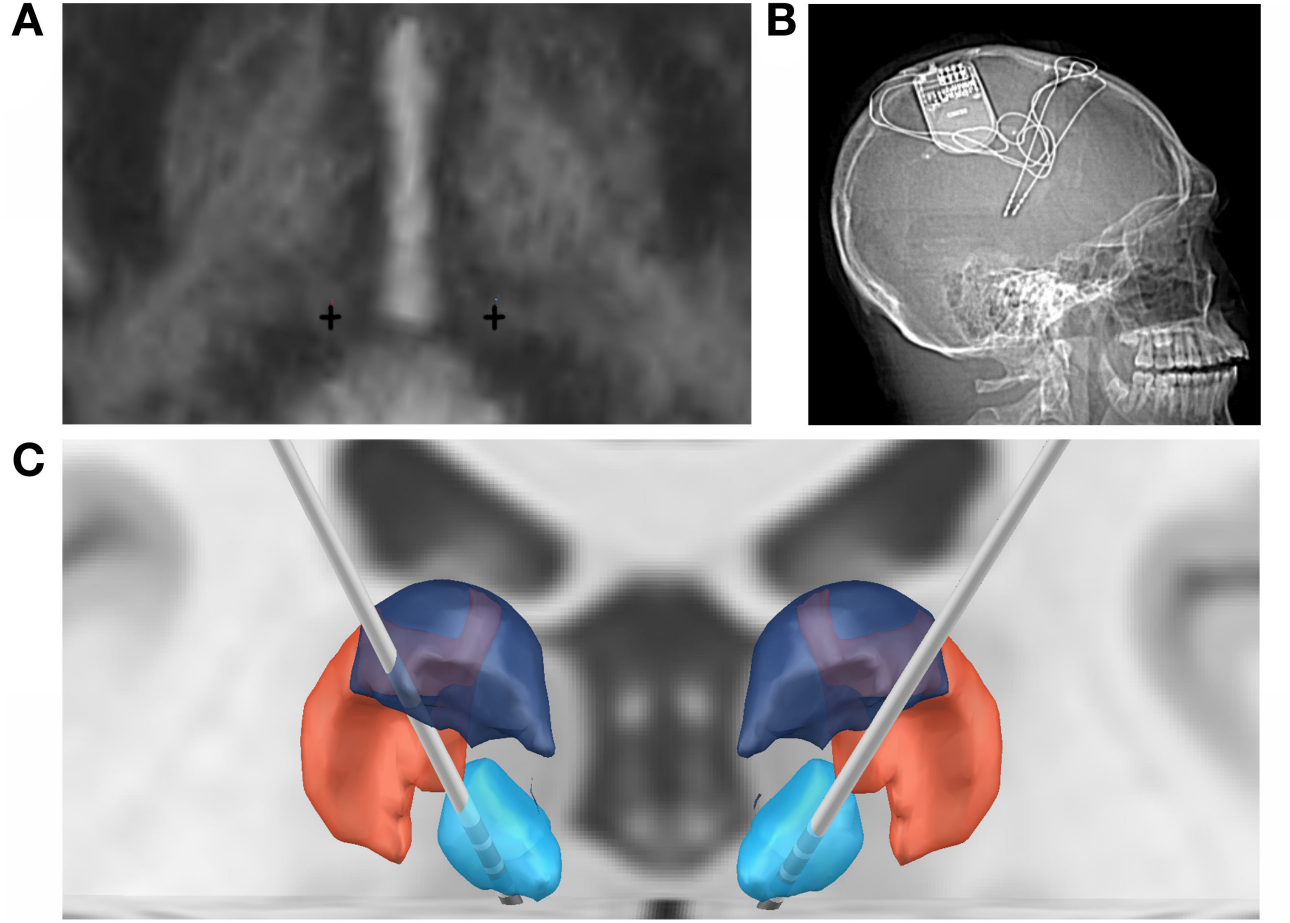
Bilateral RNS CMN implantation. (A) Direct targeting of the bilateral CMN (crosses) in AC-PC orientation. (B) Post-implant x-ray of RNS system. (C) Coronal 3D reconstruction of bilateral RNS implantation targeting the CMN (blue), in relation to the posterior part of the ventral posterolateral nucleus (VPLp; red) and posterior dorsal part of the ventral lateral nucleus (VLpd; purple).
